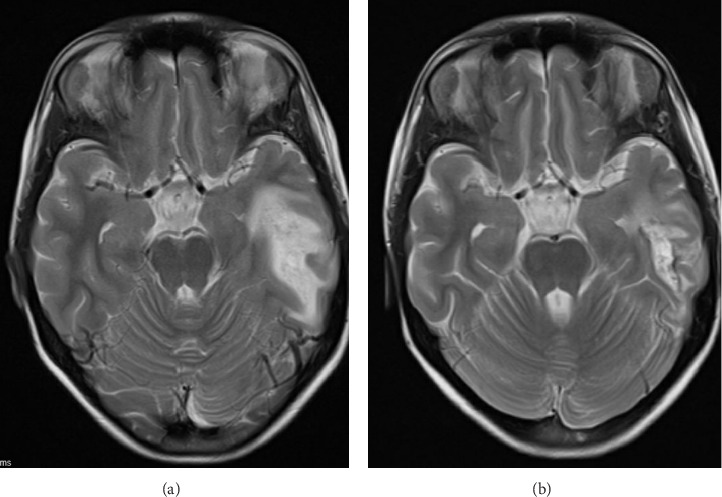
T2-weighted MRI brain taken on Day 3 and in week 4 of admission, respectively. On initial presentation (a), an area of hyperintensity was seen in the subcortical and deep white matter of left temporal lobe in T2W images. The overlying cortex in the left temporal lobe is mildly swollen with sulcal spaces effacement. The differential causes of the above radiological findings included encephalitis and demyelinating diseases. The MRI 4 weeks later (b) showed resolving T2W hyperintensity and cerebral edema after start of high dose systemic corticosteroids and intravenous immunoglobulin. However, blooming artefact was present at the posteroinferior aspect, suggestive of subacute hemorrhage product. Microhemorrhage could be a result of cerebral vasculitis. (a) On Day 3 of admission. (b) In week 4 of admission.
